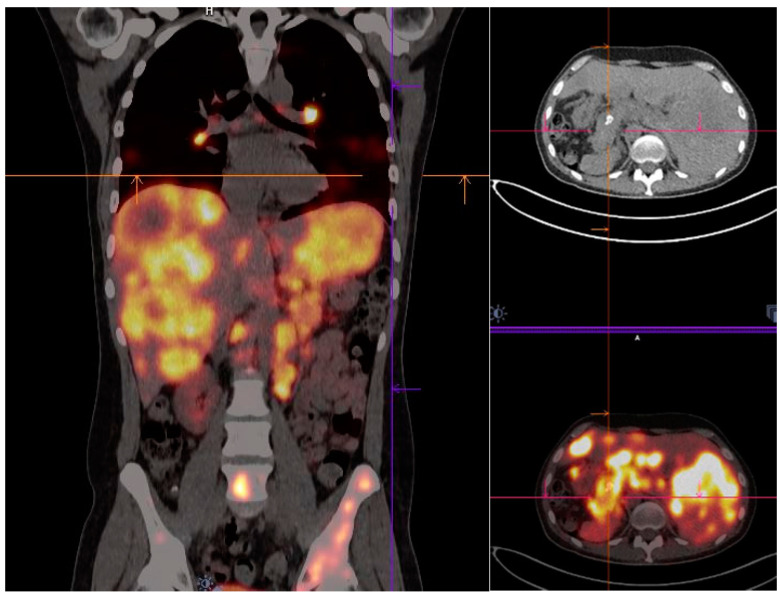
[^64^Cu]Cu-DOTA-TOC PET/CT: A 36-year-old gentleman with NET of the pancreas with Ki67 at 10%; bilobar multi-segmental multiple hepatic and multiple vertebral and pelvic bone metastases are evident in the provided coronal (left side) and trans-axial images (right side), including the liver and skeletal system.
